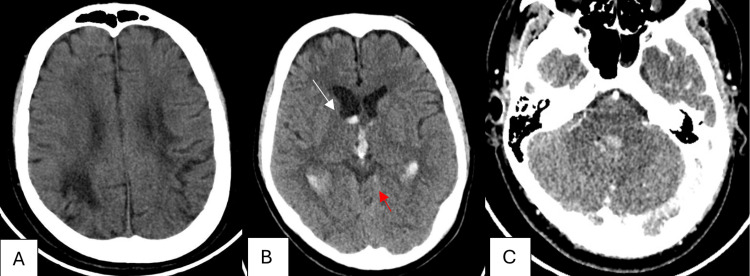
(A, B) Unenhanced brain CT and (C) contrast-enhanced brain CT showing IVH (white arrow) with minimal SAH (red arrow), along with previously known right and left parietal infarctions SAH: subarachnoid hemorrhage; IVH: intraventricular hemorrhage; CT: computed tomography
